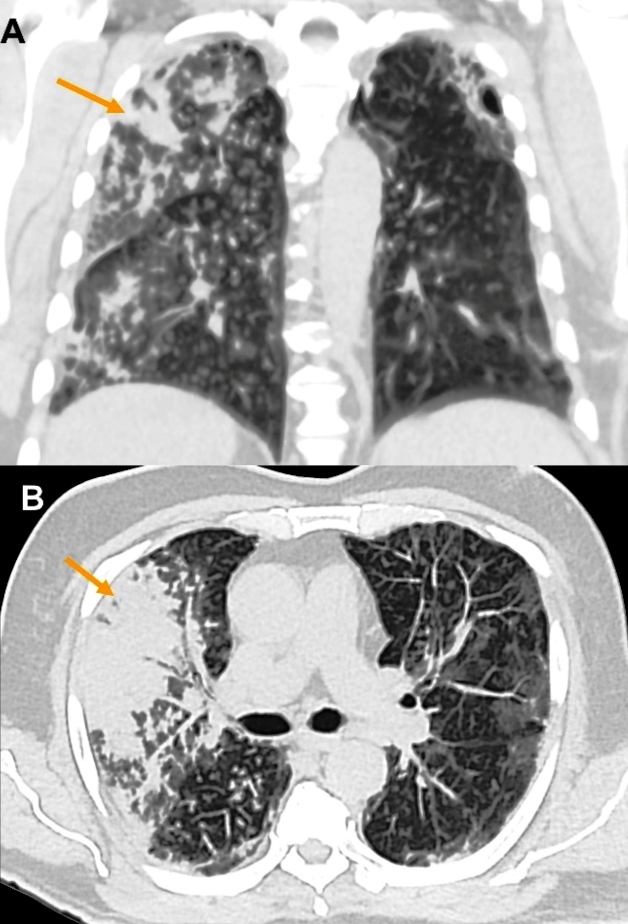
Chest CT in lung window, coronal (A) and axial (B) slices, show multiple
